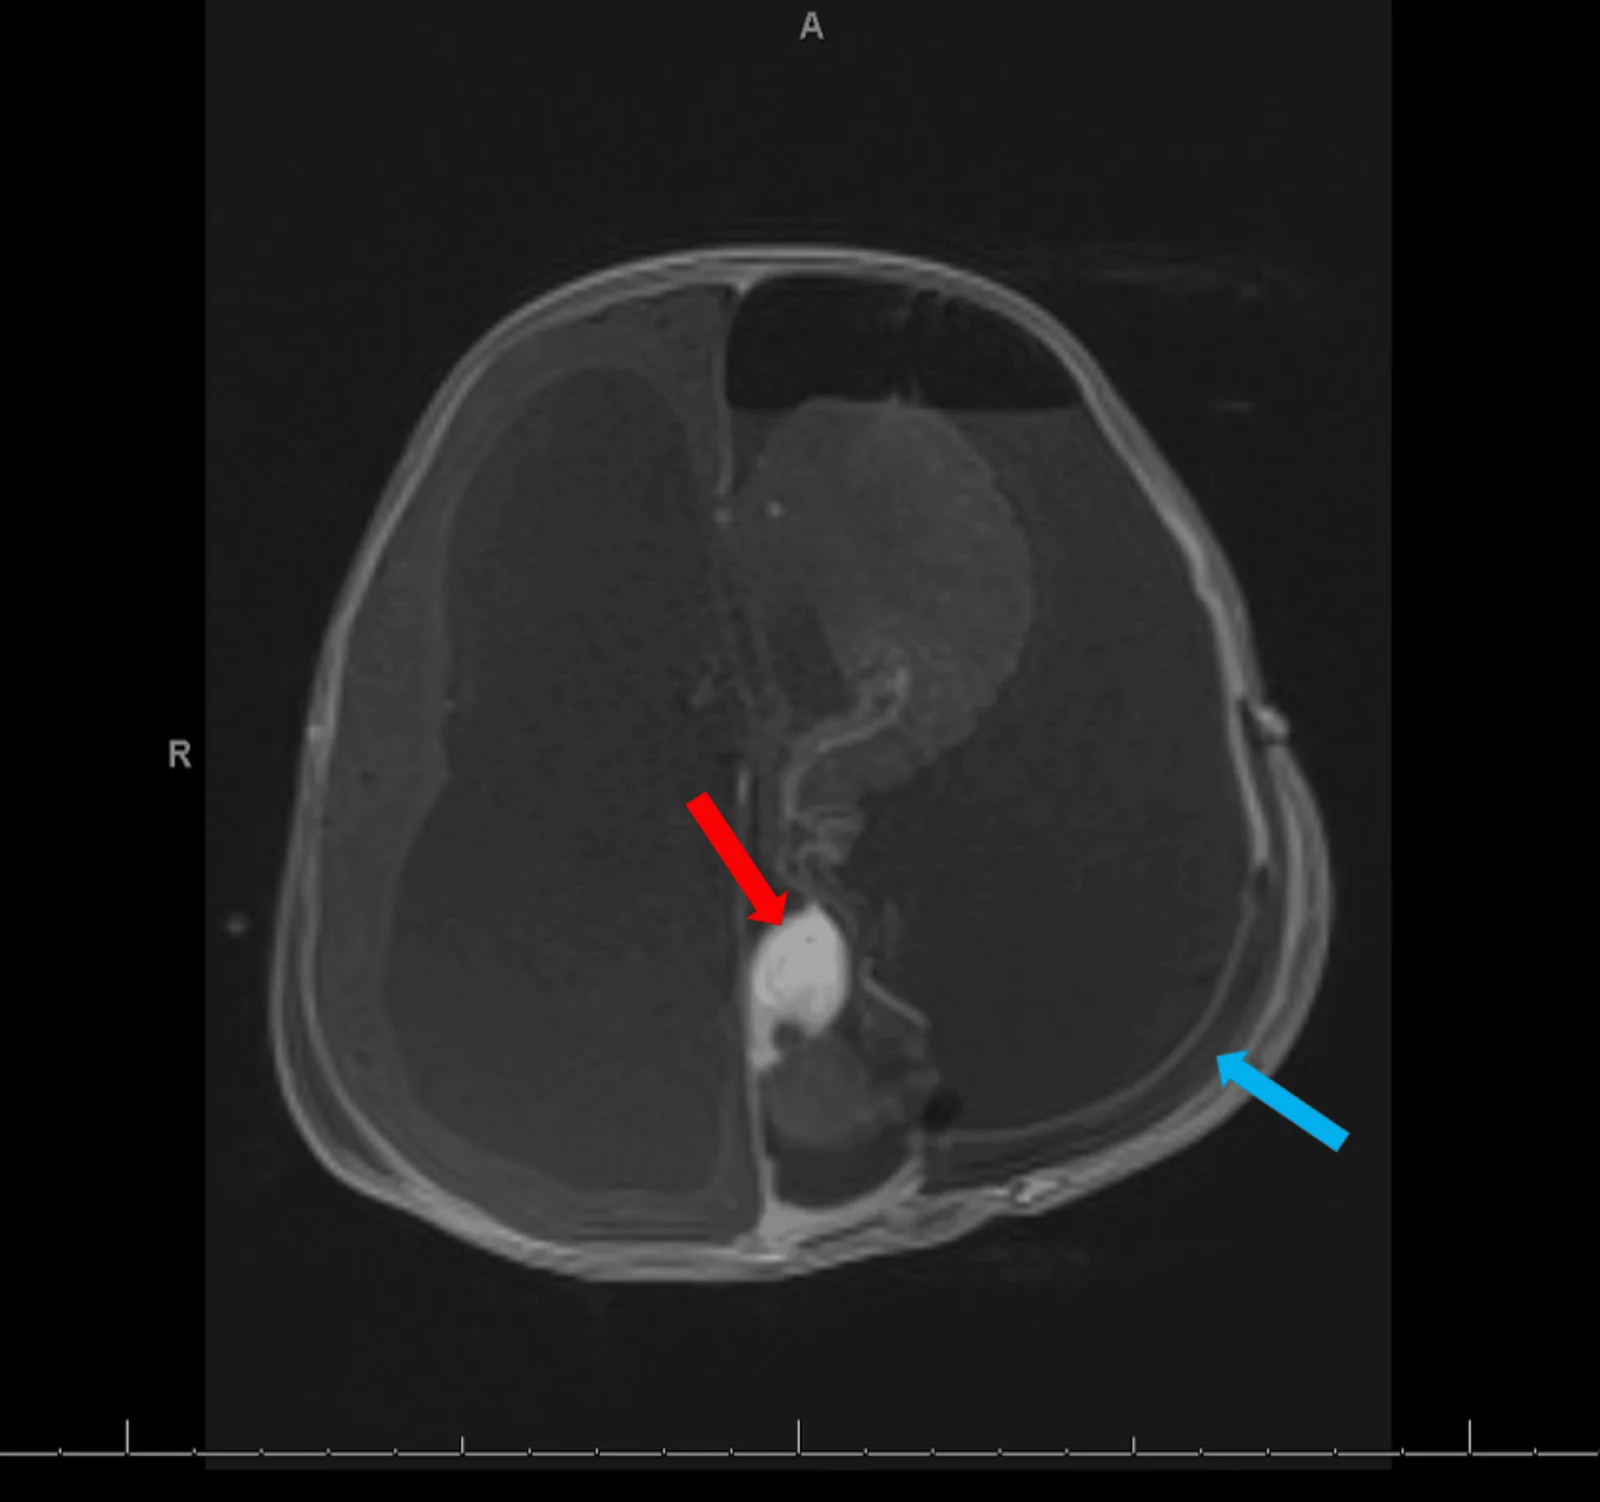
Immediately post-operative MRI (T1 post-contrast). Post-operative change with hematoma around the resection bed (red arrow) and an extradural fluid collection (blue arrow). No evidence of residual lesion identified.
